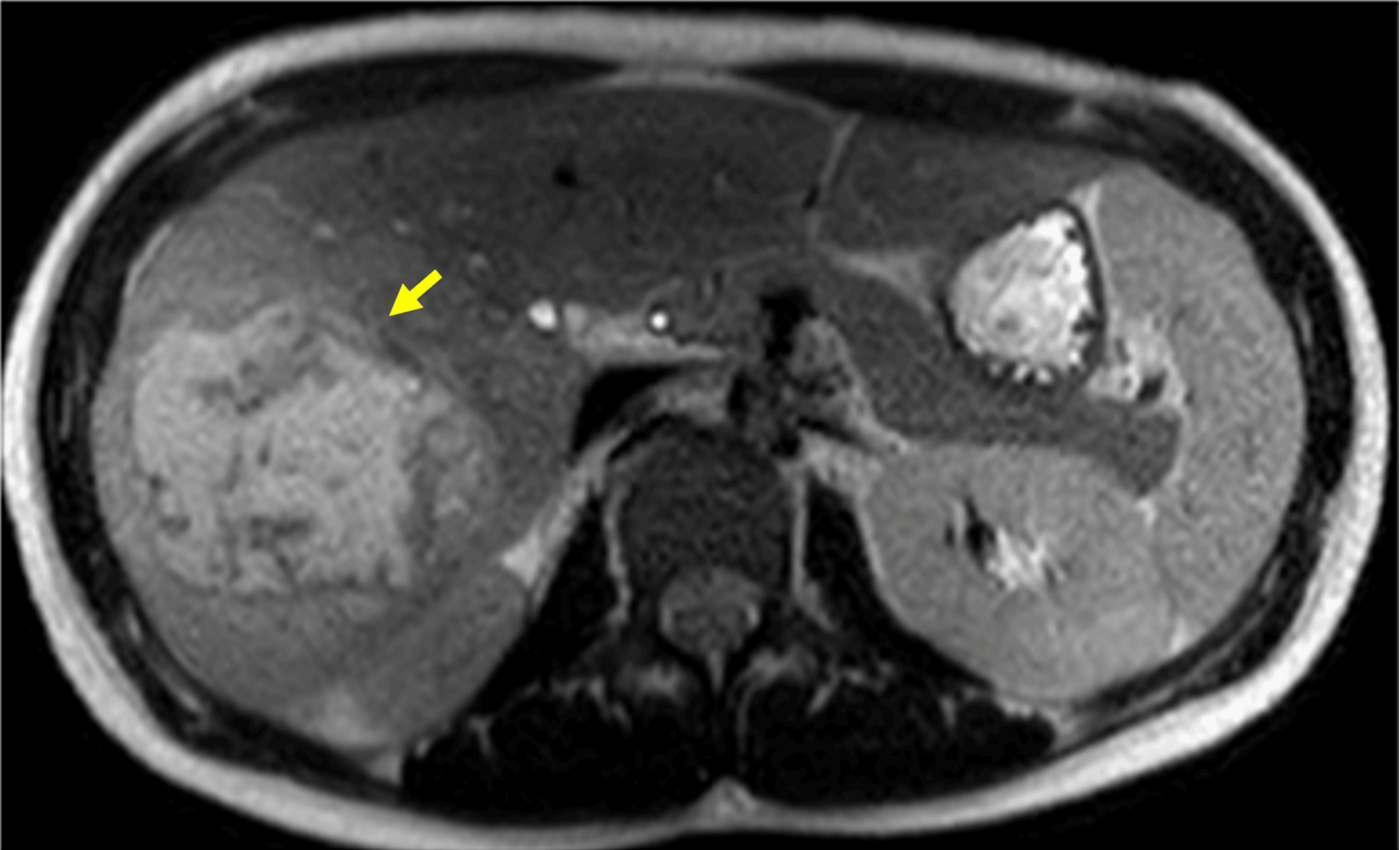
Abdominal MRI with findings of a large, complex and septated collection in the right lobe of the liver
